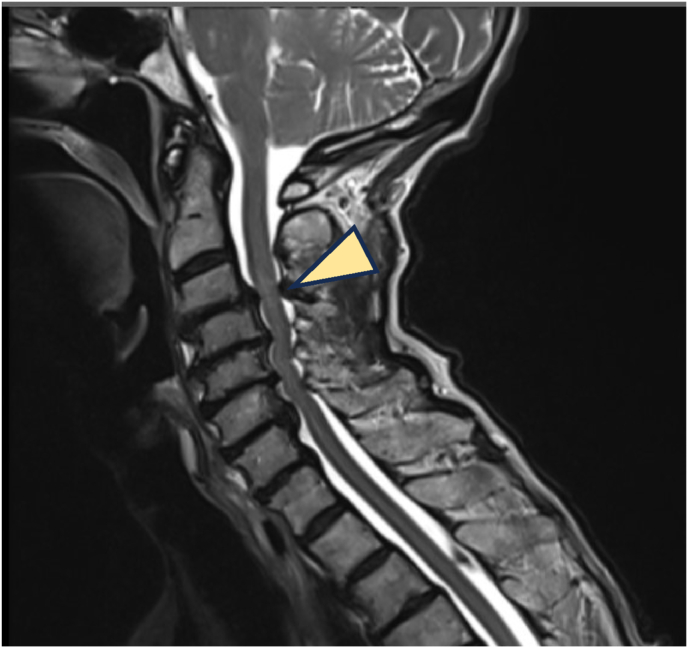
T2W MRI of the cervical spine revealed multi-level compression C3-7 and cord signal change most pronounced at C3-4 (arrowhead). After posterior cervical decompression and fusion, she showed significant improvement in arm strength and pain.
